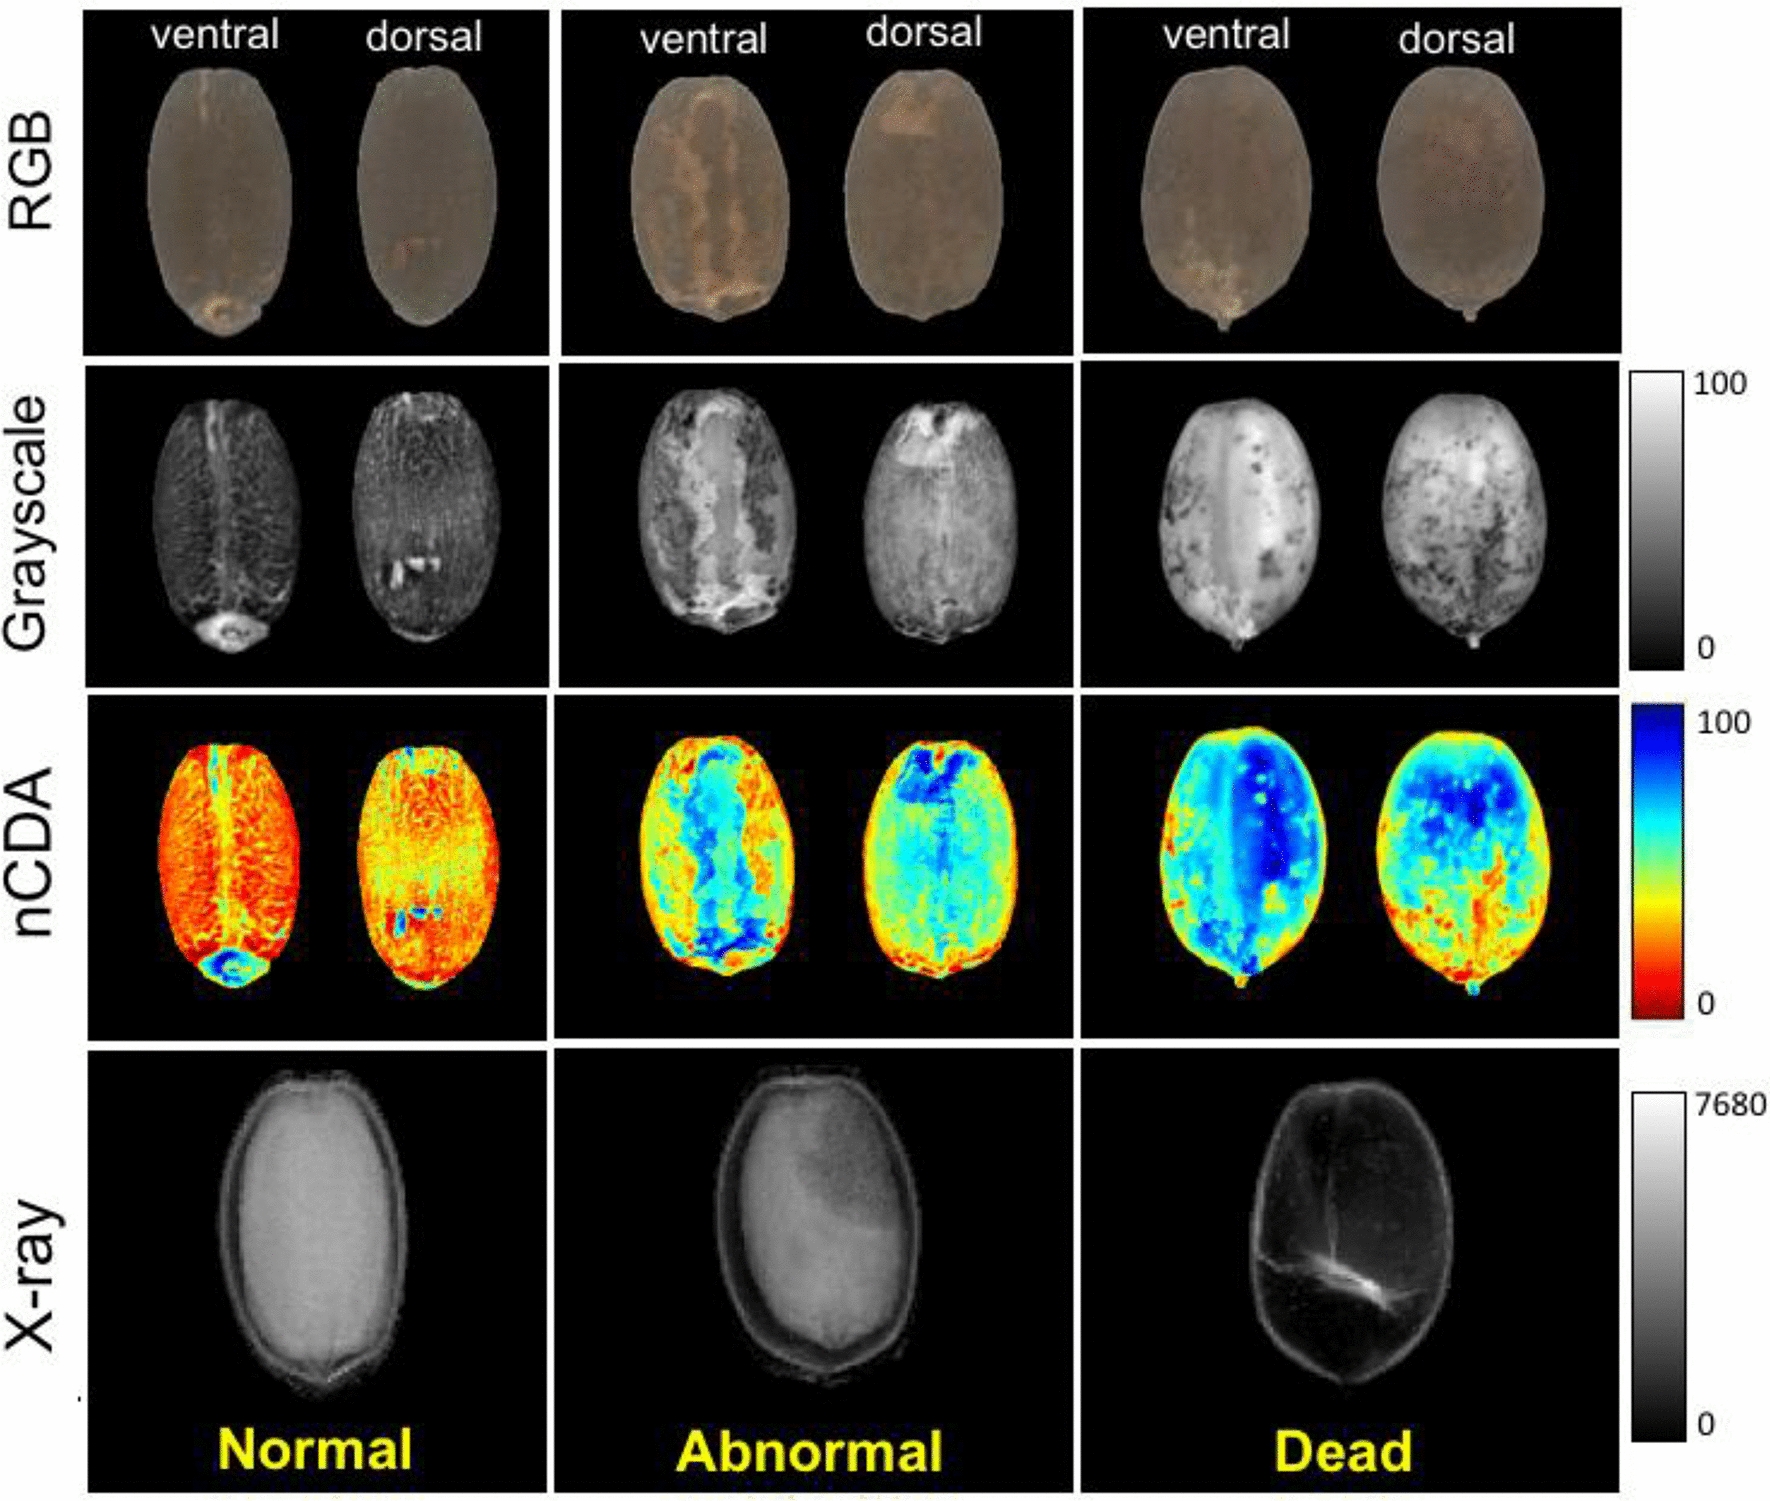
Raw RGB images of ventral and dorsal surfaces of *Jatropha curcas* seeds based on germination capacity and corresponding reflectance images captured at 940 nm (grayscale and transformed images using nCDA algorithm) and X-ray images. RGB images are represented by three-color channels (red, green and blue) to generate a single-color value for each pixel in the image. In the grayscale and transformed images using nCDA each pixel is represented by a single-value that correspond to reflectance intensity. Higher pixel values in the X-ray images indicate higher tissue density
